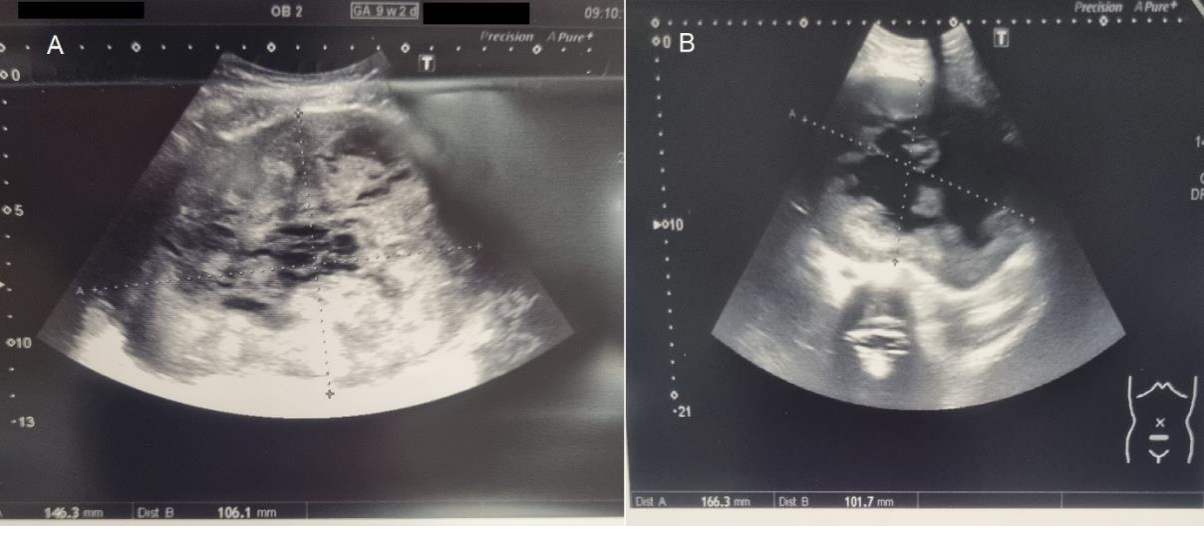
Sonographic examination of uterus fibroid. (A) Uterus fibroid at 9+2 weeks of pregnancy. (B) Uterus fibroid at 13 + 2 weeks of pregnancy.
